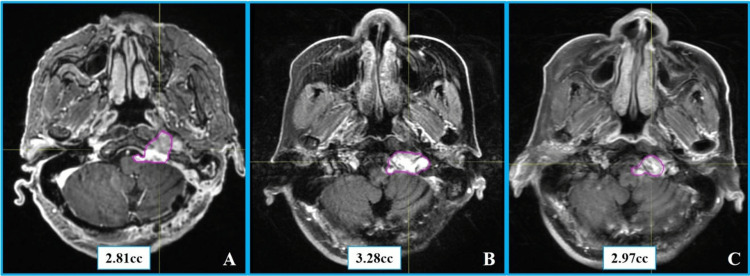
Contrast-enhanced MRI images of Case 2. (A) Axial contrast-enhanced T1-weighted imaging with contouring of the tumor volume before radiosurgery. (B) The same sequence with tumor volume contouring one year post-ZAP-X radiosurgery. (C) Axial contrast-enhanced T1-weighted imaging with tumor volume contouring three years post-ZAP-X radiosurgery.
